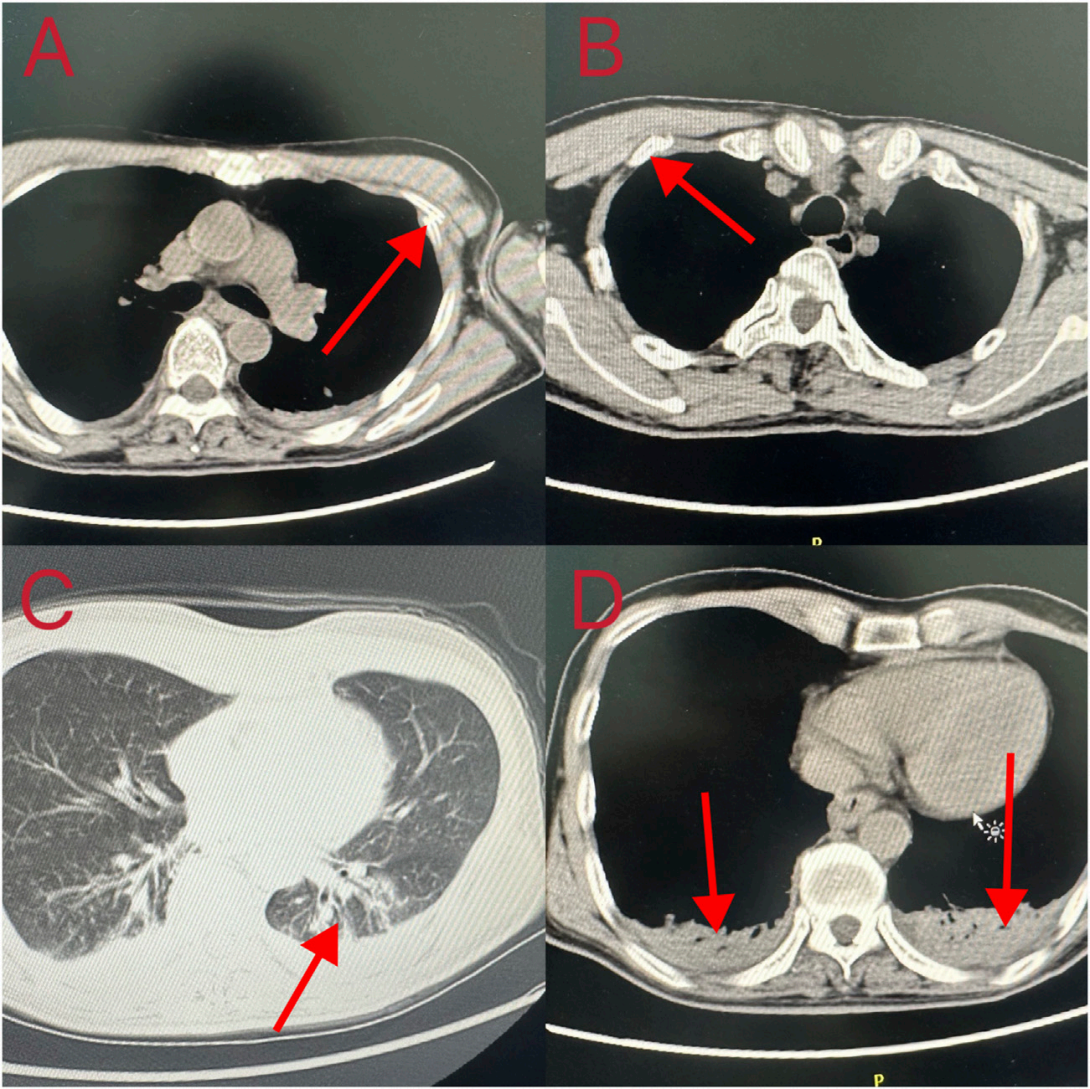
Computed tomography changes in the patient’s lungs. (A, B) Fractured ribs; (C, D) Double pneumonia and bilateral pleural effusion.
